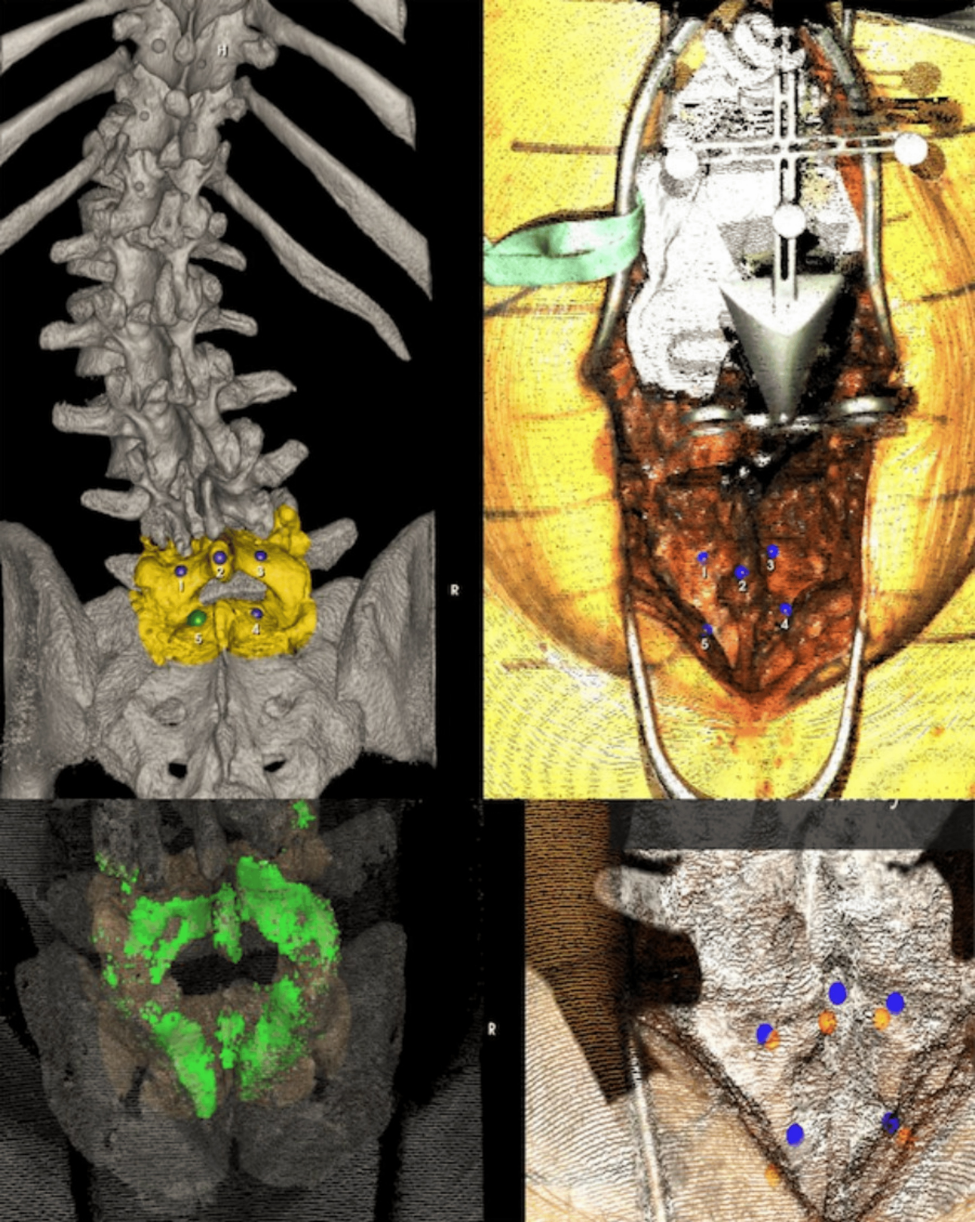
Intraoperative imaging showing the preselected fiducial points on the preoperative CT and in situ (top) at the L2-L3, along with post-registration FLASH points confirming accurate registration. Posterior column osteotomies have been performed at these levels.
